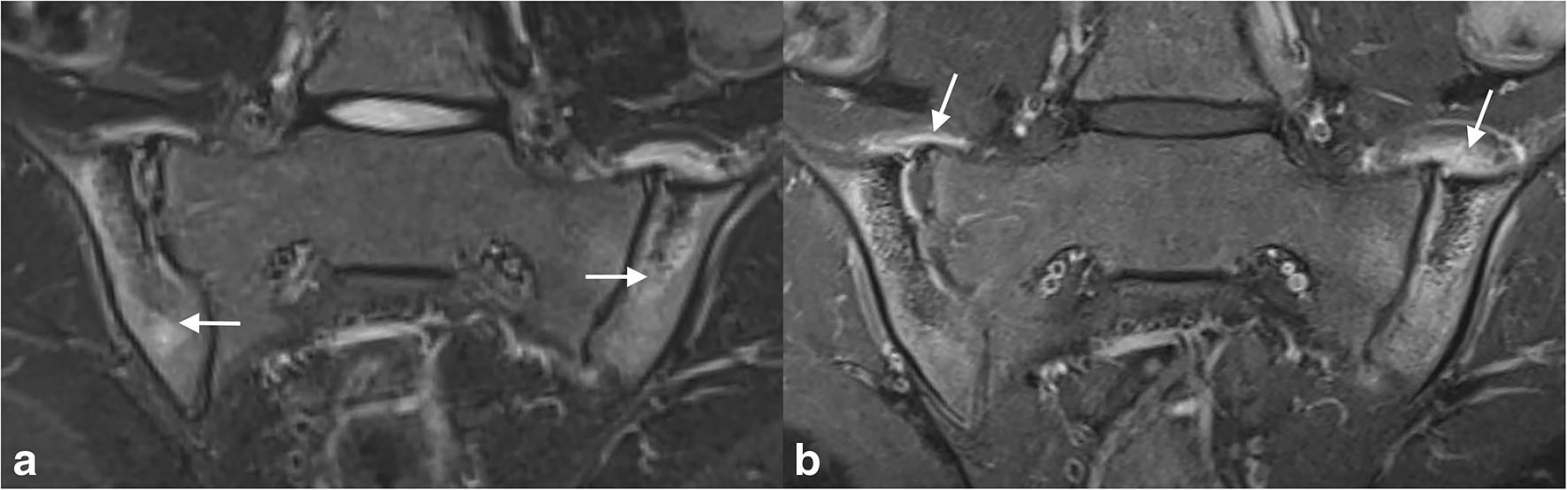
A 16-year-old boy with JIA, active sacroiliitis and an MRI showing (a) a coronal oblique STIR image showing bone marrow oedema (arrow) in, predominantly, the iliac side of the sacroiliac joints and capsulitis and (b) a coronal oblique T1-weigted fat-saturated post-Gd image showing bone marrow enhancement, joint enhancement and capsulitis (arrow). In this case, capsulitis can be seen as hyper-intense T2-weighted signal with enhancement at the T1-weighted fat-saturated post-Gd image at the cranial site of the sacroiliac joints
